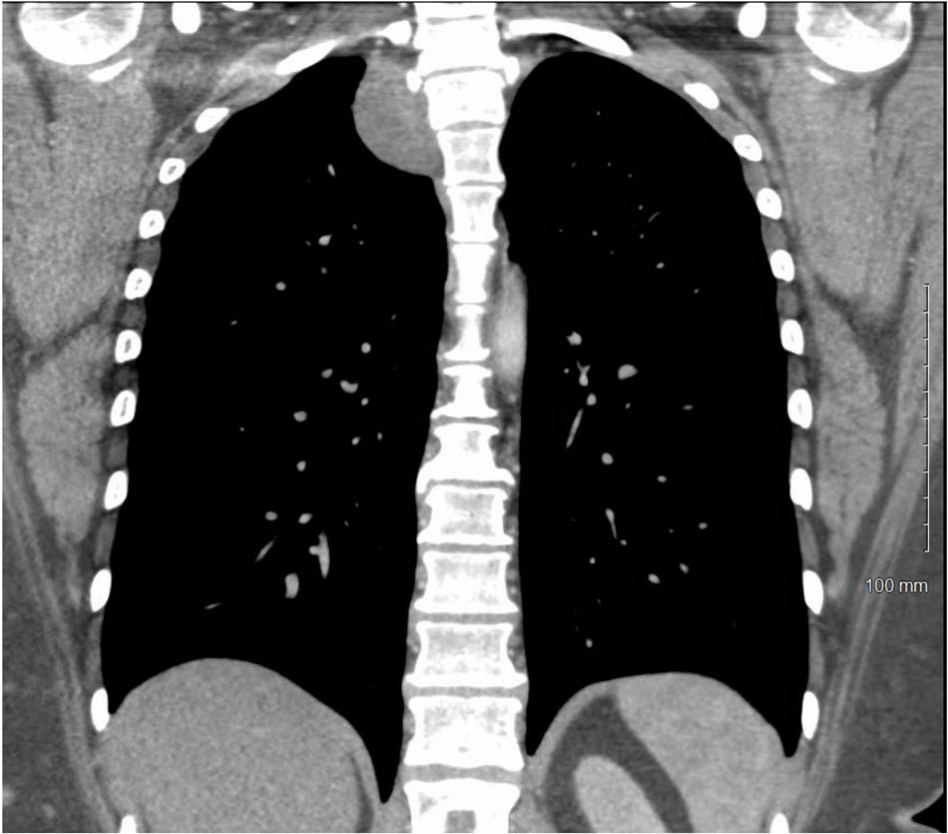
CT chest with IV contrast coronal view of right T2-T3 paravertebral mass.
